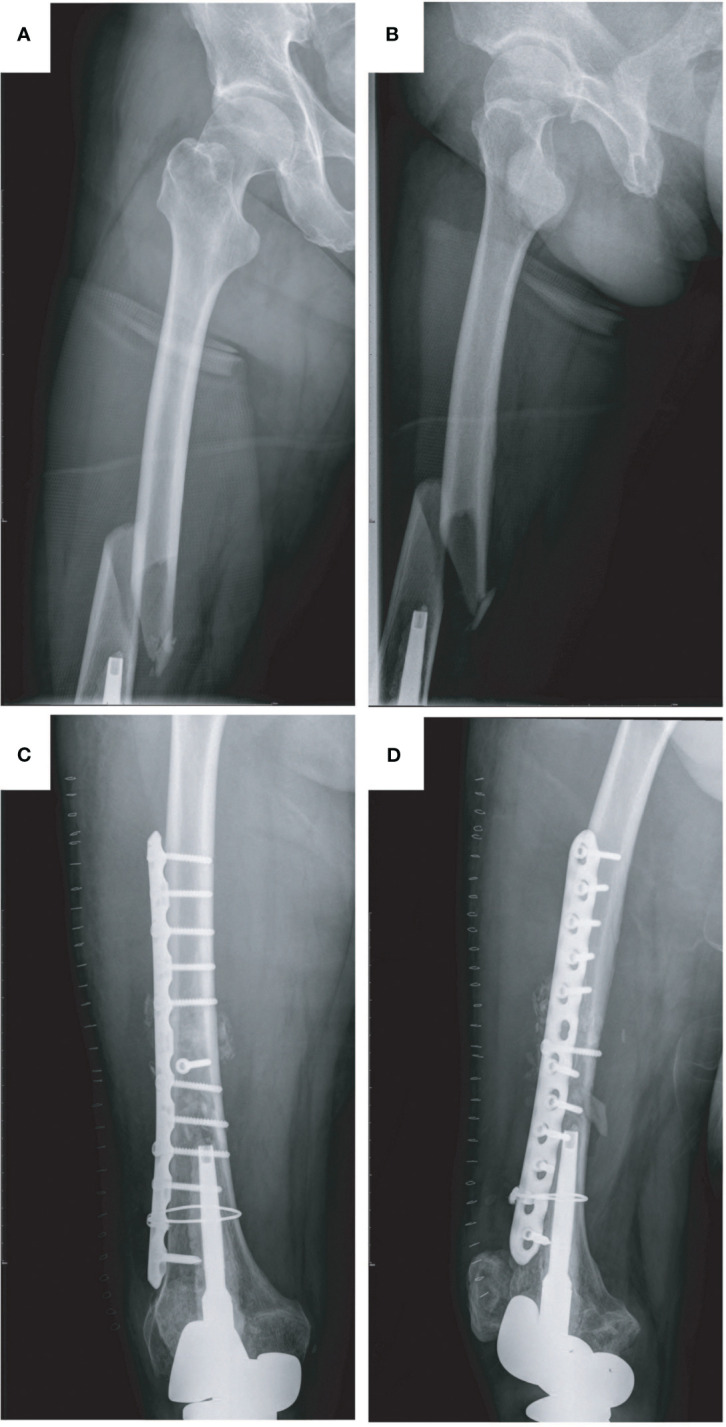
Type II periprosthetic fracture and corresponding surgical strategy (open reduction and internal fixation with plates and screws, reinforcement with wire rope). (A, B) Preoperative X-ray image of the right femoral fracture and (C, D) postoperative X-ray image of the right femur.
